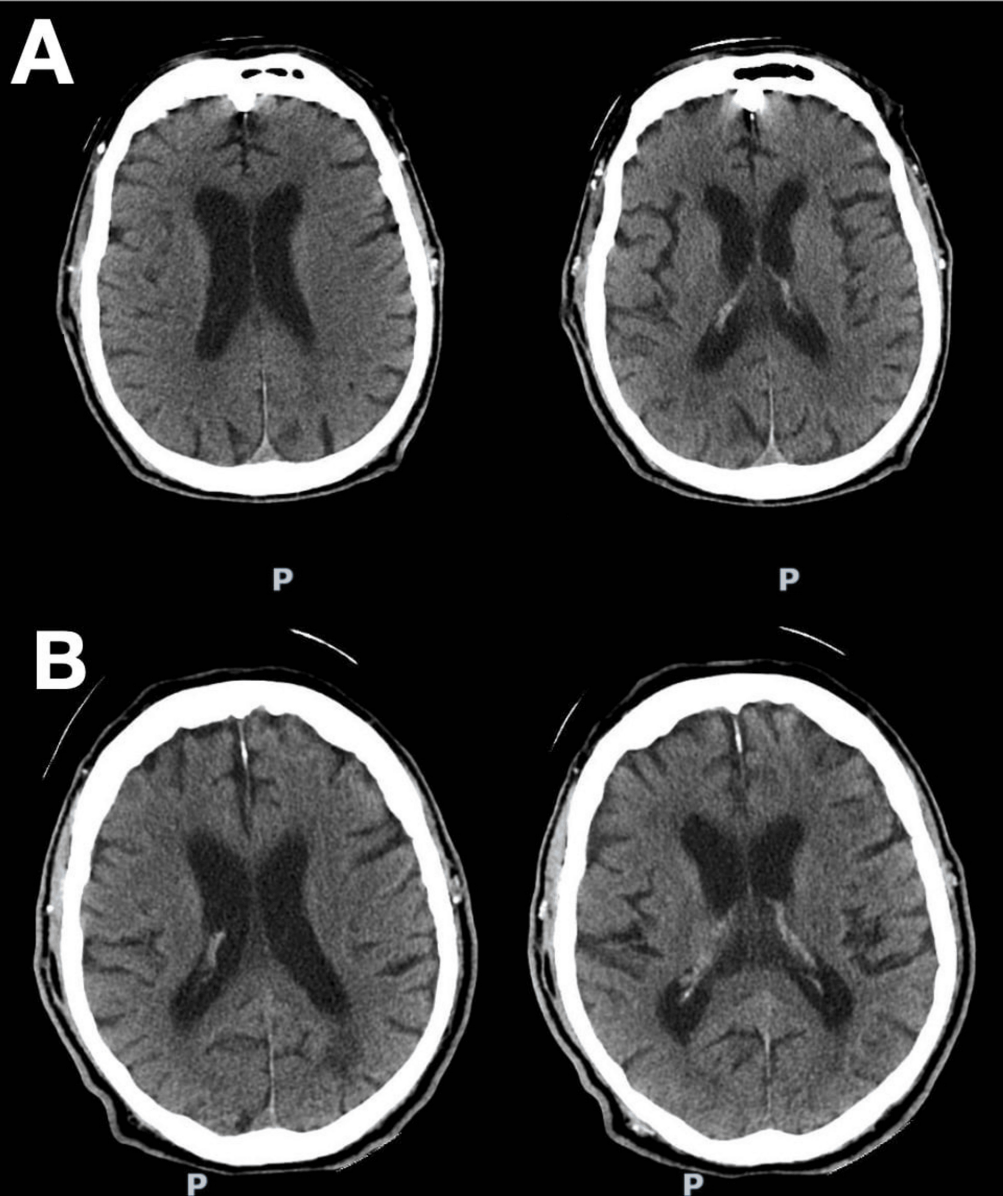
Non-contrast brain CT scans (A) Initial scan with no evidence of hemorrhage or acute ischemia. (B) Follow-up at 48 hours showing no ischemic lesions. CT: computed tomography
